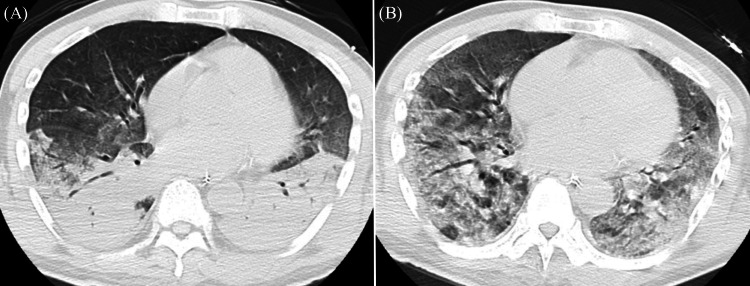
CT of the chest (A) On day 6, computed tomography (CT) of the chest showed bilateral alveolar infiltrates, suggesting the exudative stage of acute respiratory distress syndrome (ARDS). (B) On day 13, CT showed bilateral interstitial infiltrates, suggesting the proliferative stage of ARDS. CT, computed tomography; ARDS, acute respiratory distress syndrome
